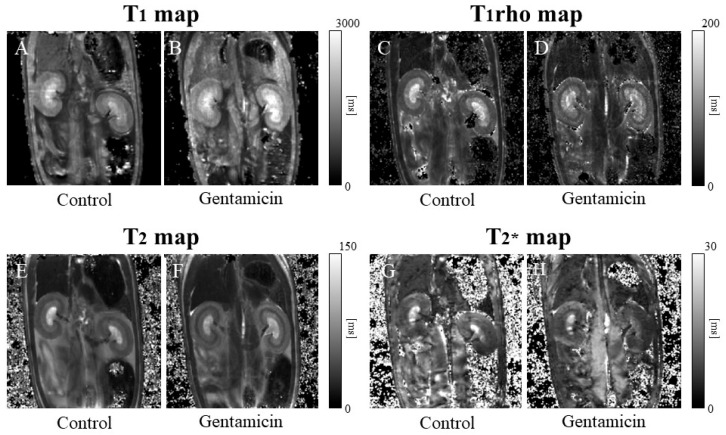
(A–H) Magnetic resonance imaging of the T_1_, T_1rho_, T_2_, and T_2_* maps. (A,B) The T_1_ map shows a kidney T_1_ value increase in the gentamicin model (B). (C,D) The T_1rho_ map did not show significant changes in T_1rho_ values. (E,F) The T_2_ map shows increased T_2_ values due to kidney injury, and the outer stripe of the outer medulla was visible (F). (G,H) The T_2_* map did not change significantly in the gentamicin model (H). The scale bar on the T_1_ map shows 0 ms to 3000 ms. The scale bar on the T_1rho_ map shows 0 ms to 200 ms. The scale bar on the T_2_ map shows 0 ms to 150 ms. The scale bar on the T2 star map shows 0 ms to 30 ms.
